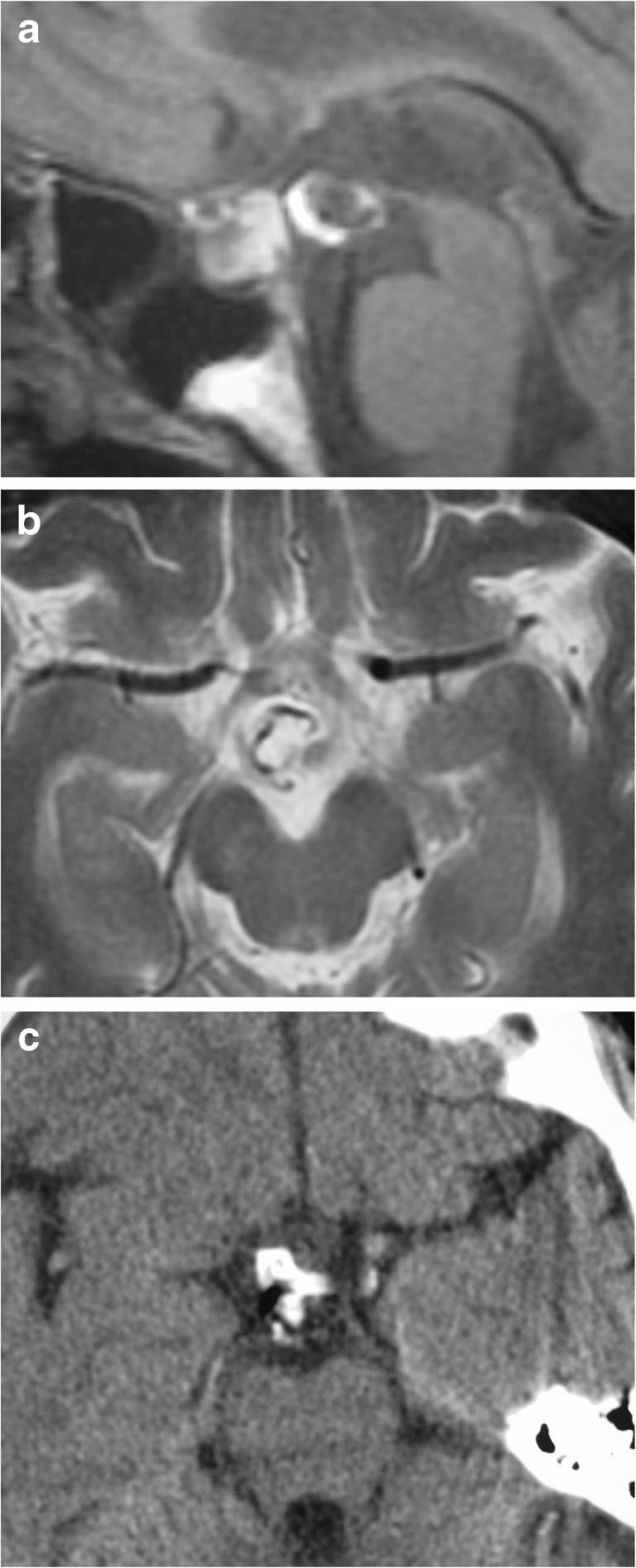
Teratoma. Sagittal T1WI (a) and axial T2WI (b) show a lesion at the undersurface of the hypothalamus which has mixed low, intermediate and high signal. Axial CT image (c) shows the lesion to contain zones of calcification, fat and intermediate soft-tissue attenuation
